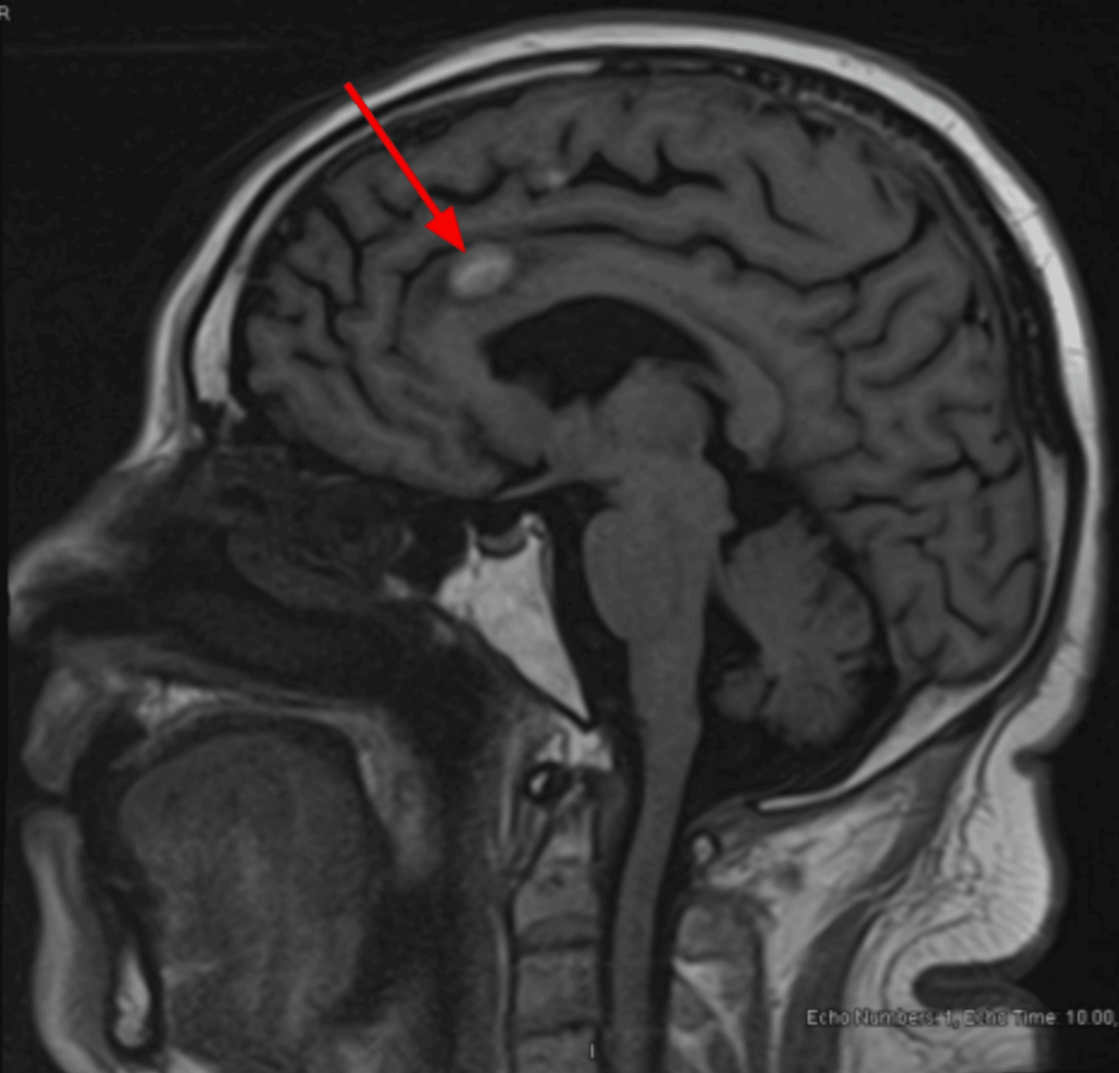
Brain MRI (September 2024) showing stability of metastases
